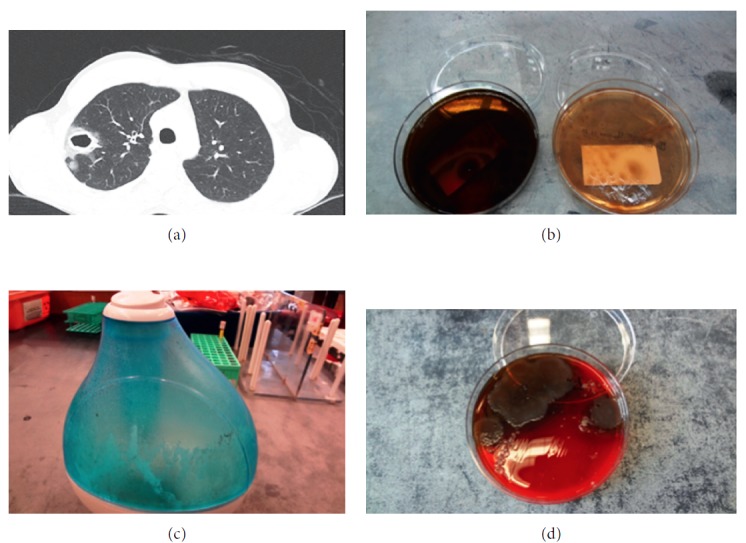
(a) Cross section of CT chest showing a right upper lobe cavitary lesion. (b) Respiratory cultures plated on blood and soy agar. (c) Humidifier with film inside water basin. (d) Humidifier culture on blood agar showing colony growth with pigment.
